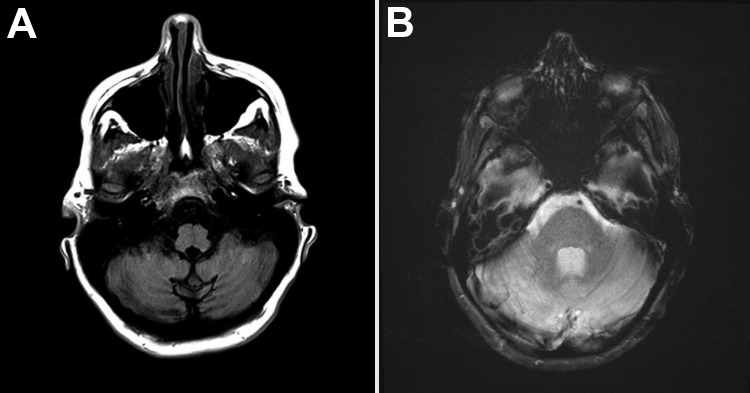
A) Magnetic resonance images of the brain of a woman with cerebellitis associated with influenza A(H1N1)pdm09, United States, 2013. T1-weighted axial MRI brain sequence showing hypo-intensity of bilateral cerebellar hemispheres. B) T2-weighted axial MRI brain sequence showing hyperintensity of bilateral cerebellar hemispheres.
